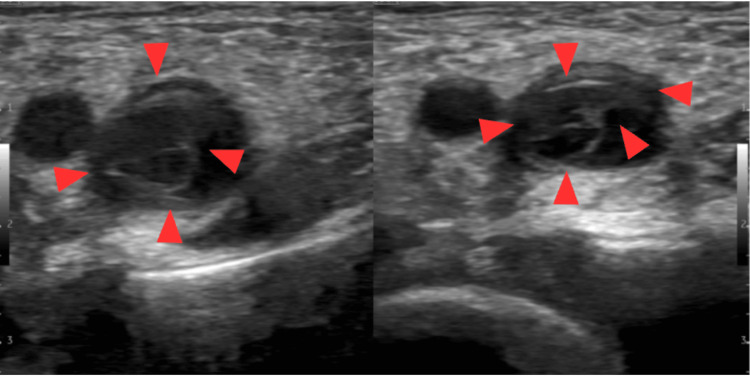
Transverse (short-axis) ultrasound image of the femoral vein demonstrating intraluminal thrombus (red arrows) extending from the common femoral to the popliteal vein.
